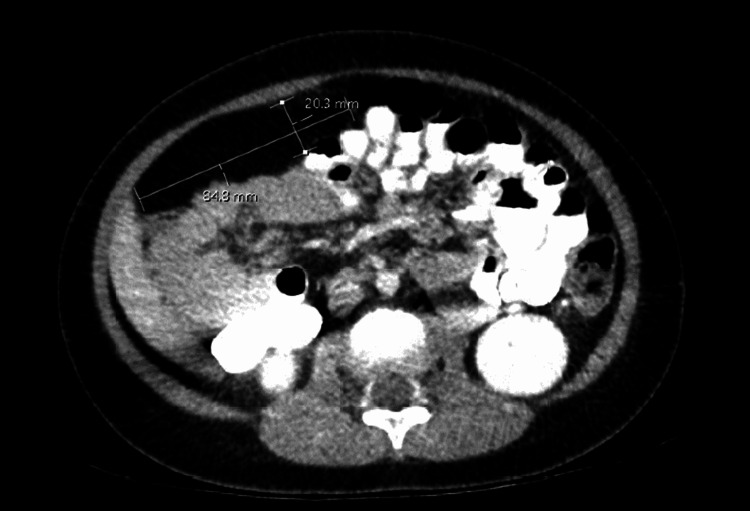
Initial CT abdomen and pelvis – image 1 Focal area of fat in the right upper quadrant adjacent to the inferomedial aspect of the liver measuring 8.5 x 2.0 cm with a mild displacement of the adjacent bowel. This mass raised suspicion of a lipoma CT: computed tomography
